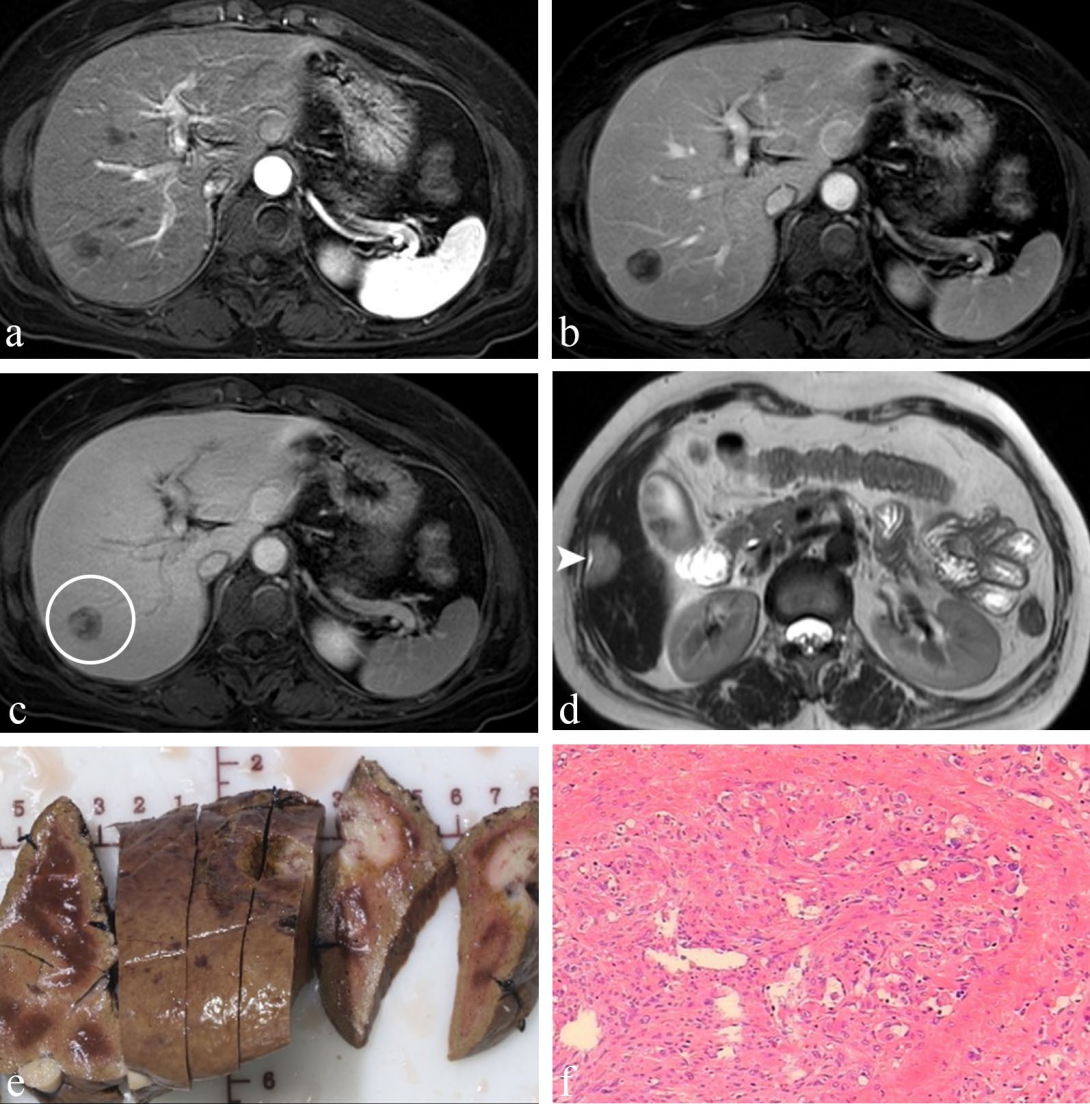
A 53-year-old woman was diagnosed with HEHE on both imaging and pathology. a–c On axial contrast-enhanced MRI, the tumour in segment 6 of the liver represents a centripetal progressive filling with a decrease in signal intensity and finally shows a targetoid appearance on the equilibrium phase (*arrow,*
